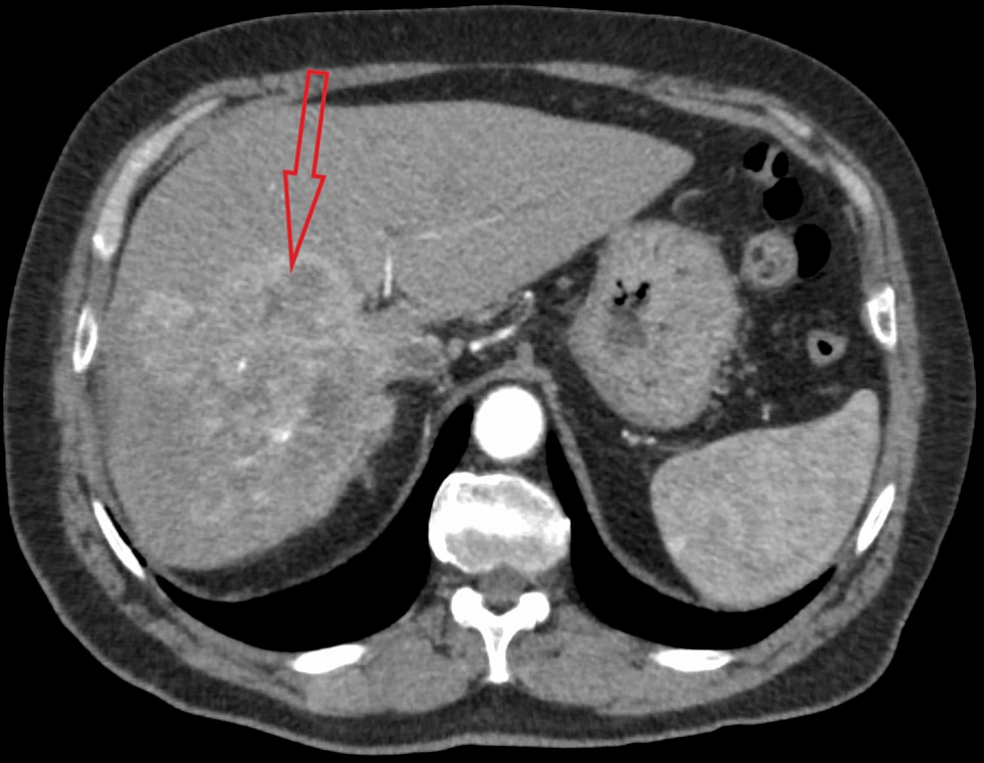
CT image of a tumor in the axial plane in the arterial phase (the red arrow points to the right liver lobe tumor) CT: computed tomography
